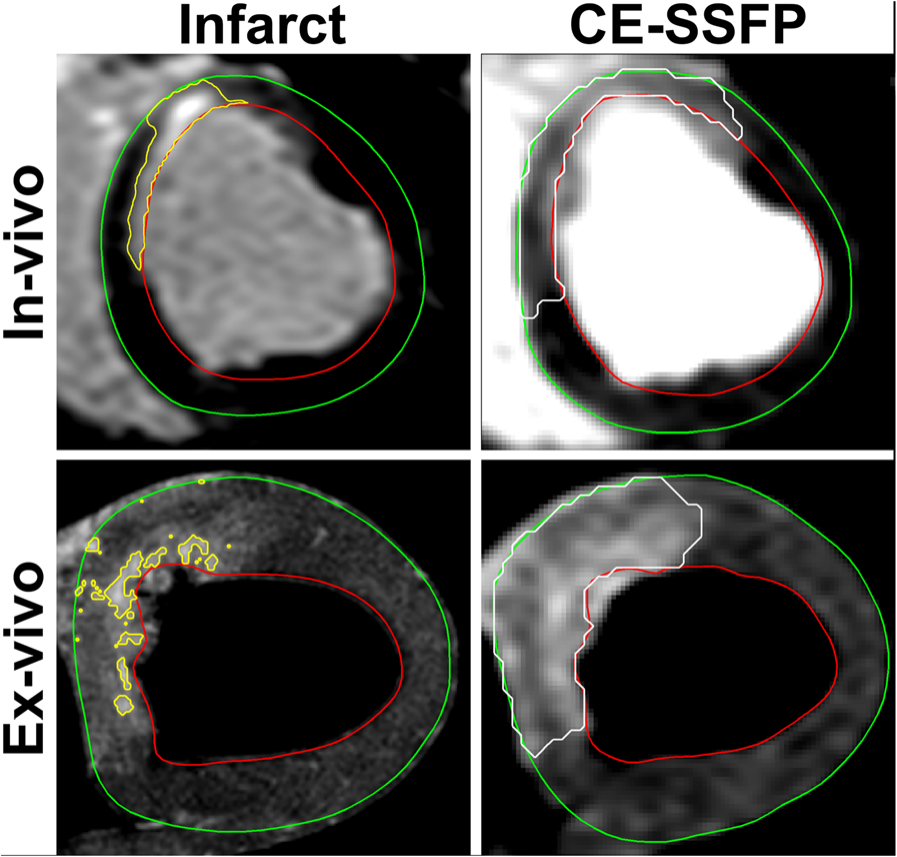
Infarct and myocardium at risk (MaR) in vivo and ex vivo. The upper row shows infarct by late gadolinium enhancement (LGE) and MaR by CE-SSFP in vivo and the lower row shows infarct by high-resolution T1-weighted imaging and MaR by CE-SSFP ex vivo. Note the significantly larger MaR compared to infarction both in vivo and ex vivo
